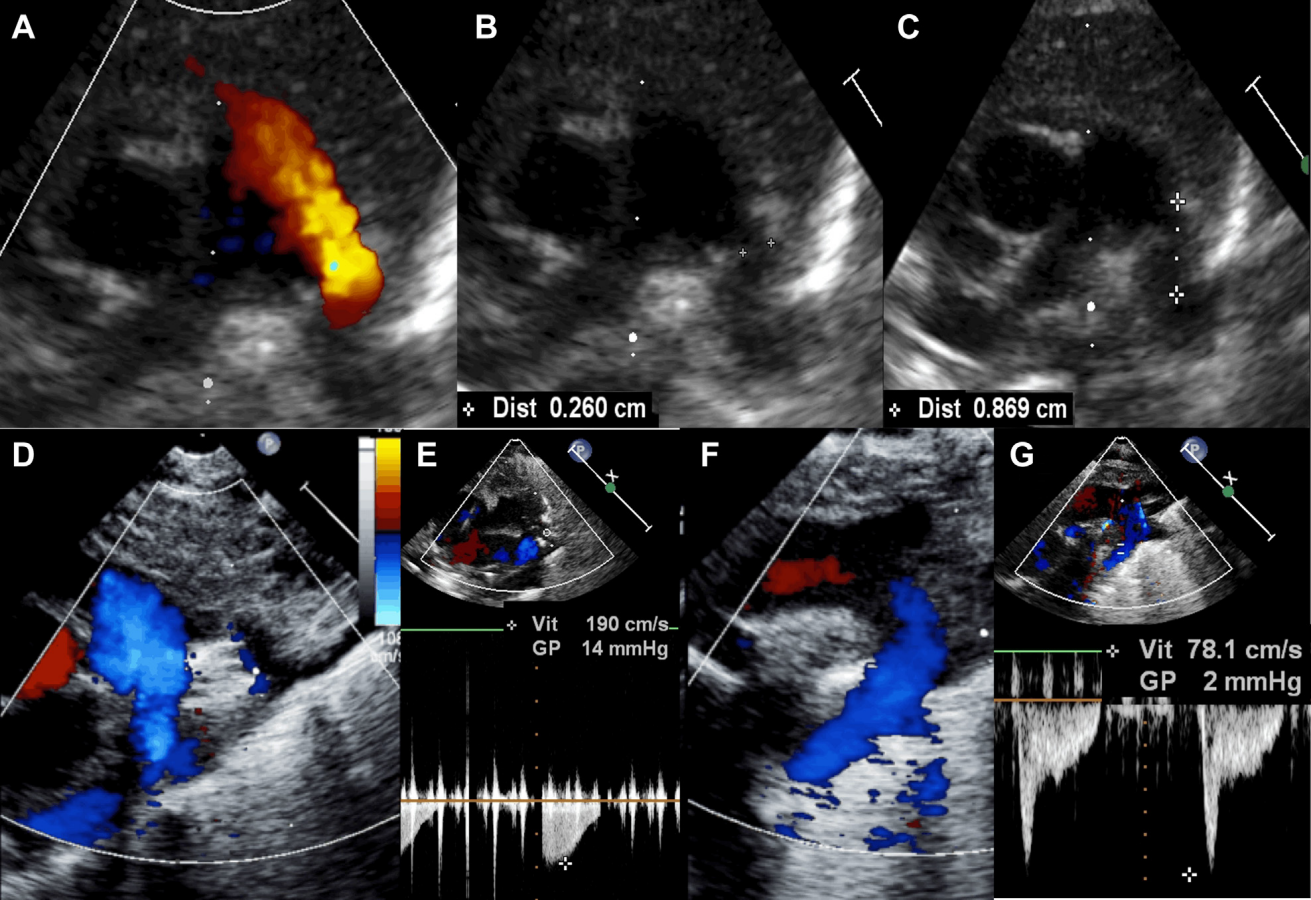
Ultrasound Guidance of Transcatheter PDA Closure in ELBW Infants (A to C) Last-Minute pre-procedural echocardiography. In the catheterization laboratory, before starting the case, a last-Minute echocardiography defines the best ultrasound window and carefully measures the ductal length and the minimal ductal diameter. Here is a large, left-to-right shunting, patent ductus arteriosus in an ex-24 weeker premature infant, with a procedural weight of 740 g (A). The minimal ductal diameter is measured at 2.6 mm (B), with a ductal length of 8.7 mm (C). (D to G) Real-time echocardiographic guidance. Ultrasound check of a well-positioned 4/2 Amplatzer Piccolo Occluder device, with no residual shunt (D and F) and no device-induced left pulmonary artery obstruction (D and E) (Vmax 1.9 m/s) or descending aorta obstruction (F and G) (Vmax 0.8 m/s). Note that the procedure is performed in an infant on high-frequency jet ventilation.
